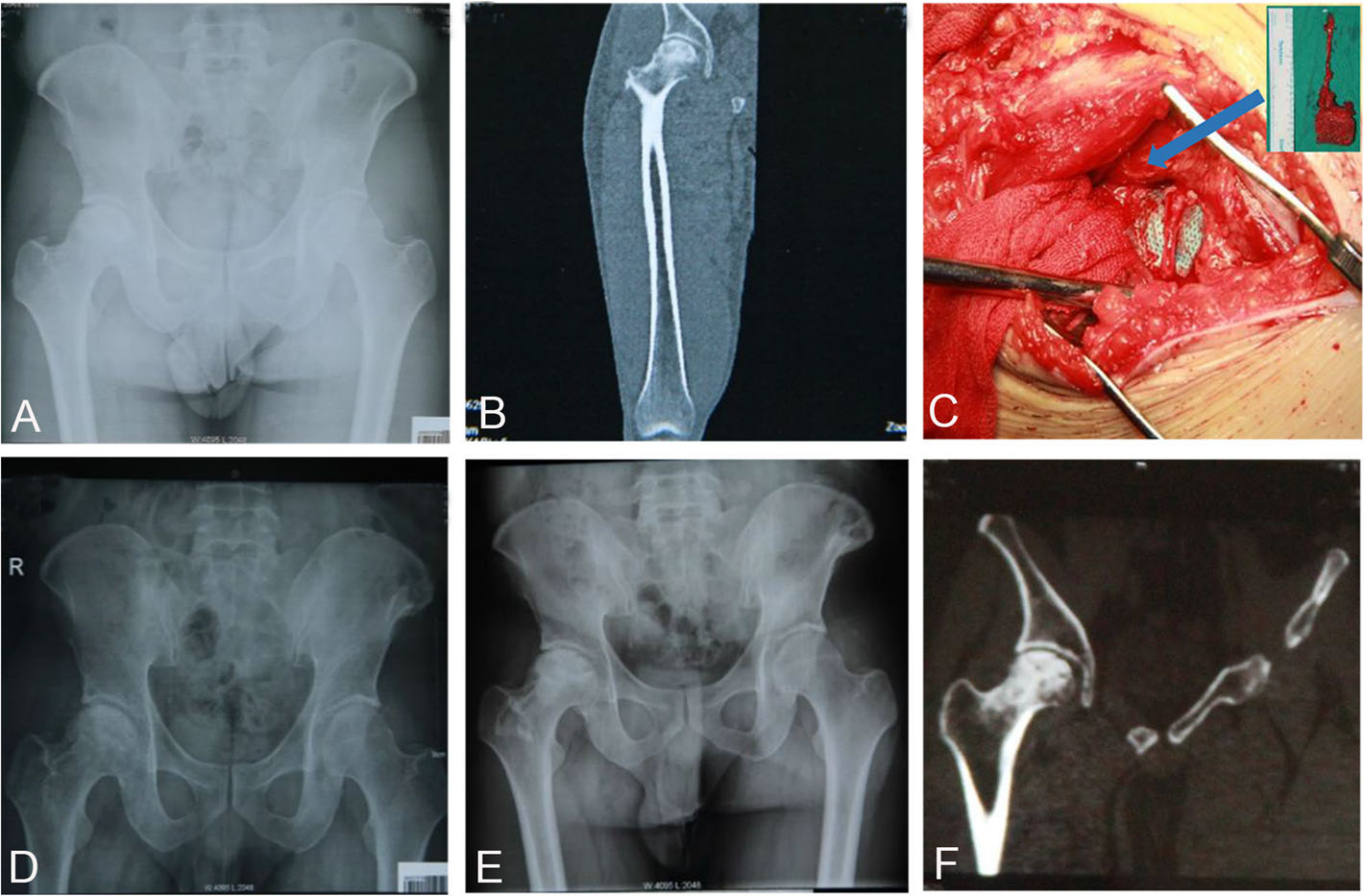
A 33-year-old male patient underwent free graft of vascularized iliac bone flap based on deep circumflex iliac vessels for treatment of idiopathic ONFH of the right side. a Preoperative X-ray and b preoperative CT scans showed heterogeneous density of the femoral head. c The iliac bone flap was separated and implanted into the bone groove. Postoperative X-rays at d 3 months, e 10 months, and f postoperative CT scans at 10 months showed no collapse of femoral heads or narrowing of the hip joint spaces
